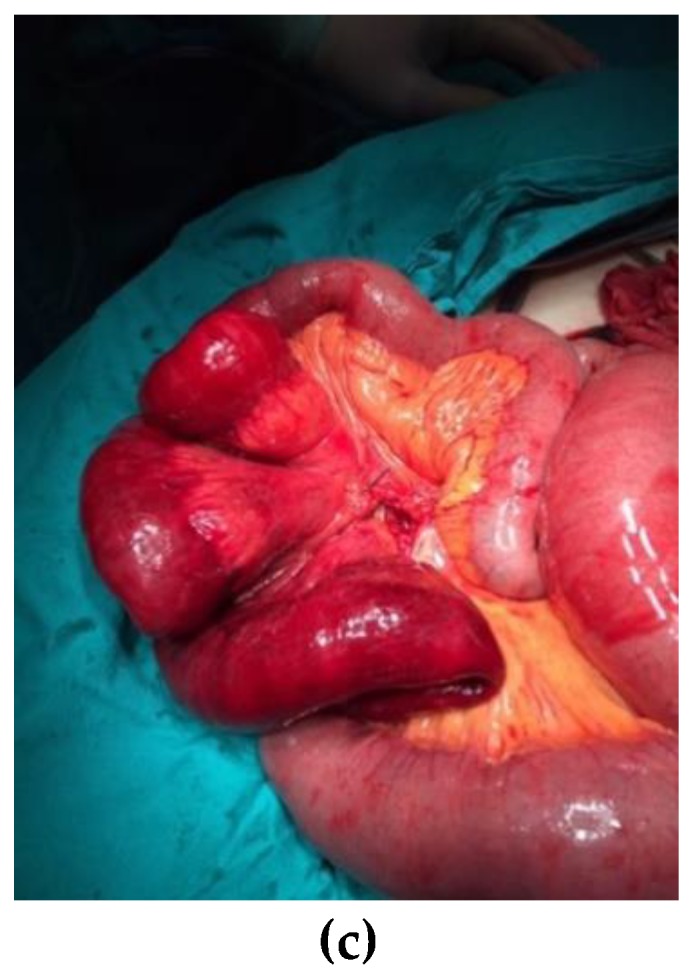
A 79-year-old male patient with previous abdominal surgery with decompensated ileus. (a) Ultrasound long axis evaluation of a fluid-filled dilated small bowel loop. Severe parietal and valvulae conniventes thickening were present, and fluid effusion adjacent was detected (*). Free fluid between bowel loops was also visualized. Peristalsis was absent. (b) CT examination with IV contrast. Fluid filled and air filled small bowel loops. The fluid filled loop appears hypoattenuating due to vascular parietal damage, and perivisceral fat stranding is present; findings were indicative of decompensated SBO. (c) At surgery, closed loops of SBO due to a bridle was evident. Vascular compromise was recognized by bluish discoloration of intestinal wall, loss of arterial pulsation, subserosal and mesenteric hemorrhage and a lack of peristalsis. After release of adhesions, bluish discoloration persisted and blocked and damaged sections of the bowel were removed.
